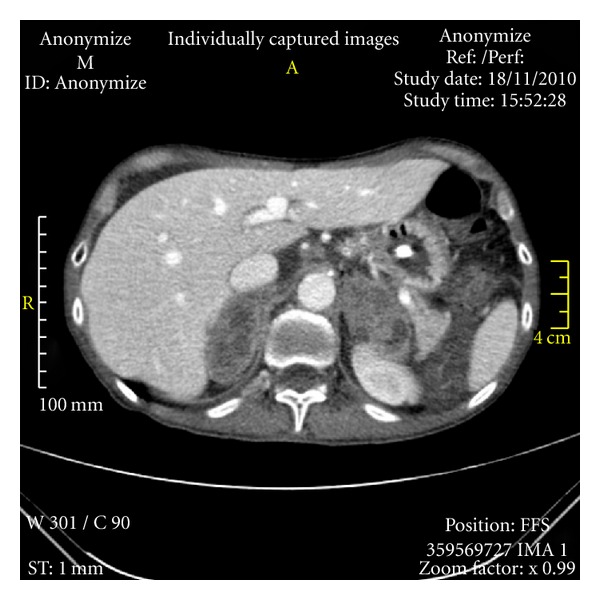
Abdominal computed tomography scan. It showed massive heterogeneous lesions in both adrenals, measuring about 5 cm in each greatest diameter.
